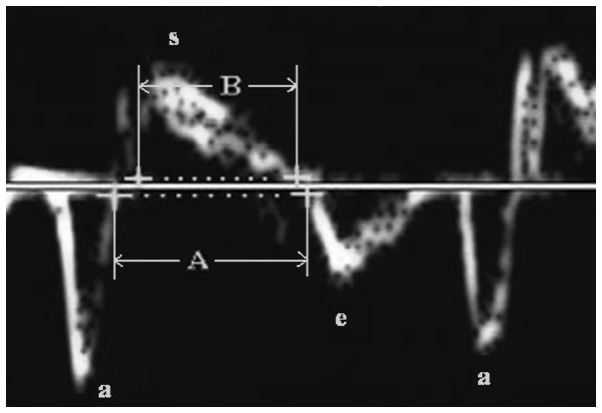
DTI wave of tricuspid annulus movement in a patient with AIDS. Lines A and B were used to calculate the Tei index. DTI, Doppler tissue imaging; AIDS, acquired immune deficiency syndrome.
